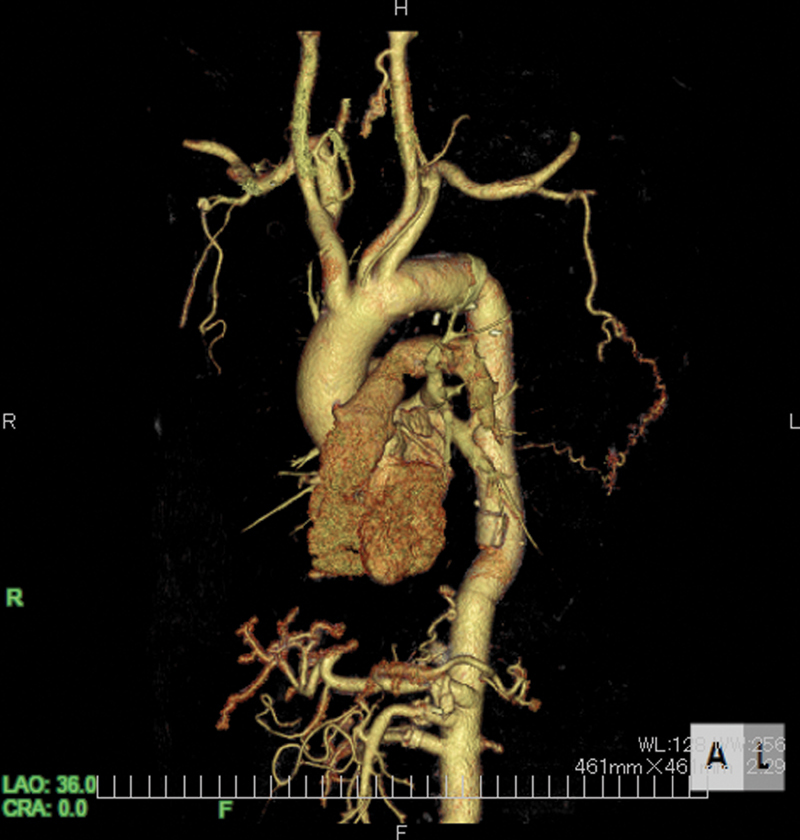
Postoperative computed tomography showing an intact collateral vessel from the left lateral thoracic artery to the Adam-Kiewicz artery.
